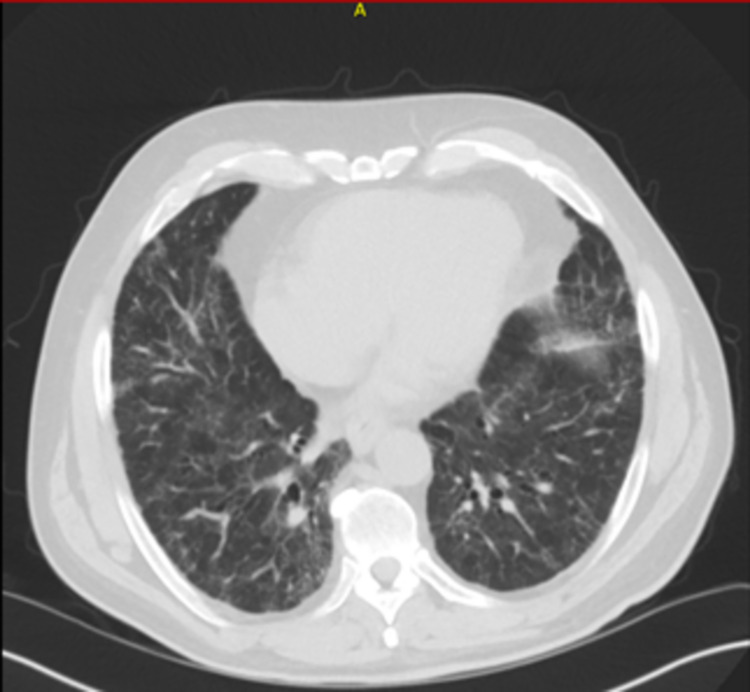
Follow-up chest CT scan six months after initiating treatment
